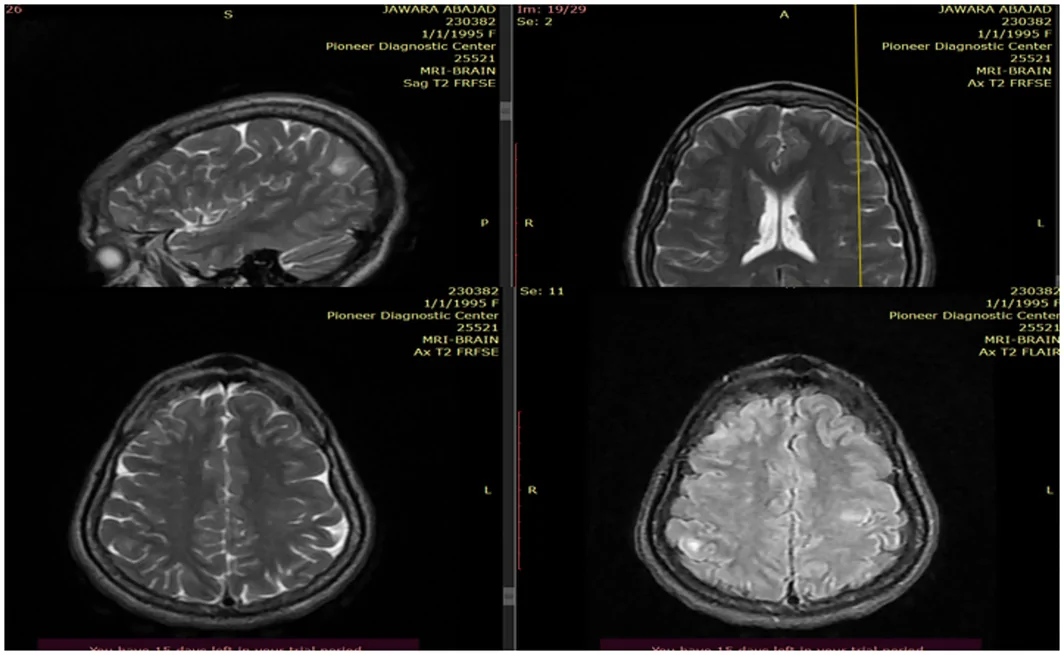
MRI of the brain: A small subependymal nodule is noted in the lateral ventricle with T1/T2 isointense signal. Multiple patchy abnormal signal changes are present in the cortical and subcortical regions of the bilateral frontal, parietal, and posterior temporal lobes, showing no contrast enhancement.
